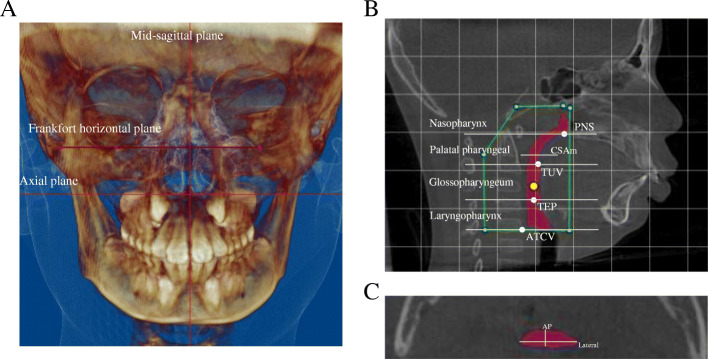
Morphologic analysis of upper airway. a Localization planes of CBCT images. b Segmented upper airway. c Minimum cross-sectional area (*CSAm*) on the axial slice of the CBCT image. *PNS* posterior nasal spine, *TUV* tip of the uvula, *TEP* tip of the epiglottis, *ATCV* anteroinferior aspect of the vertebral body of the third cervical vertebra, *AP* anteroposterior
